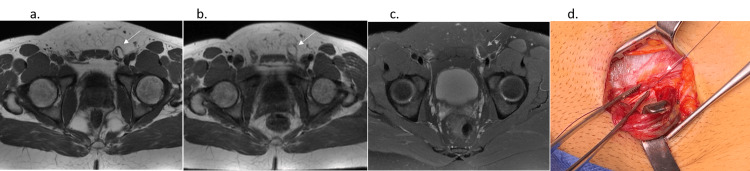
A 25-year-old man, soccer player (First League) with left pain. Axial T1 in Abdominal Wall (AW)-related Chronic Groin Pain (CGP) (a) and Axial oblique Proton Density weighted with Fat Saturation (PDFS) in AW (c) with left inguinal hernia in left inguinal orifice (white arrow) in these static MRI sequences. The important TF bulging increase in Valsalva Maneuver (VM) in axial T1 VM (b) (same patient, same level). Left AW exposed in AW-related CGP with white arrow = Prolene sutures (lowering to the inguinal ligament). The surgical clamp shows the inferior margin of an external oblique aponeurosis.
